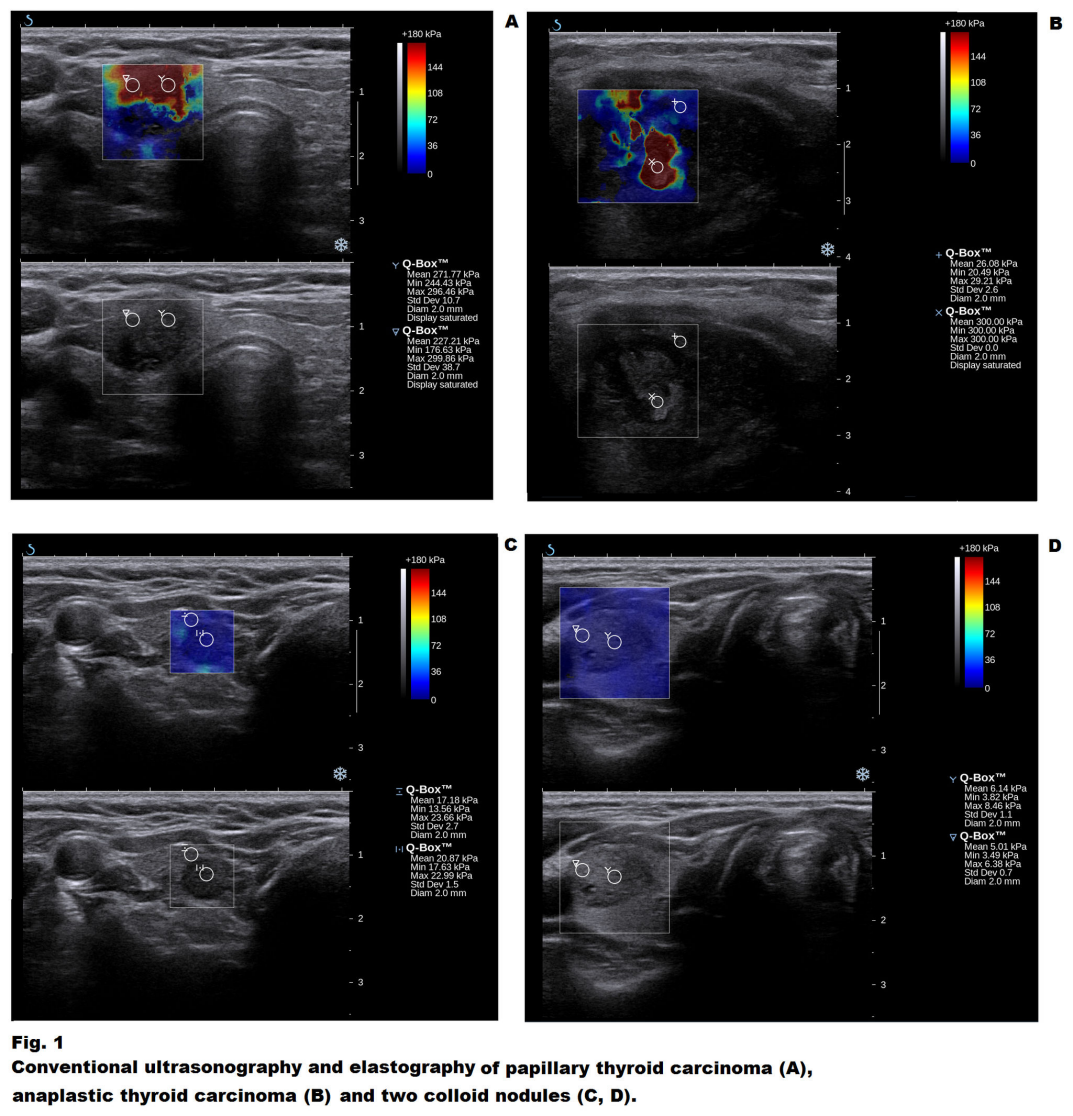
Conventional ultrasonography and elastography of papillary thyroid carcinoma (A), anaplastic thyroid carcinoma (B) and two colloid nodules (C, D).
